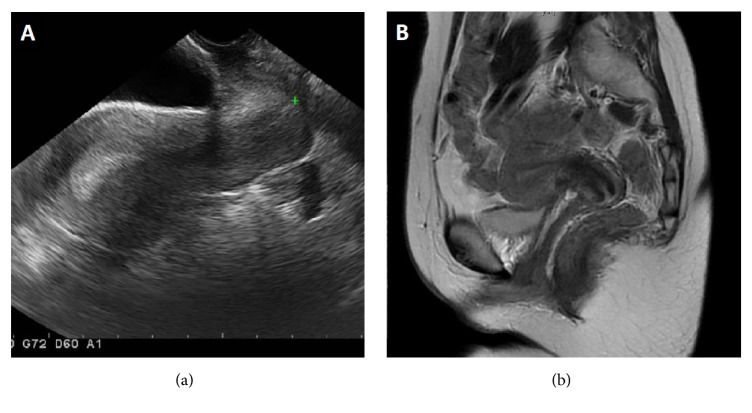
Transvaginal ultrasonography (A) and magnetic resonance imaging (B) showed a normal cervix and uterus and 2.5 cm left ovarian endometriosis, but the septum of the vagina was not detected.
